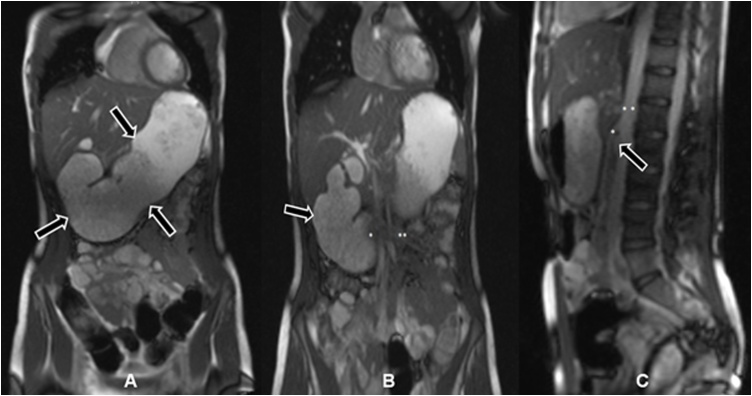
Abdominal MRI showing (A) a dilated stomach (arrows), (B) a dilated duodenum (arrow) with change in caliber from before (*) to after () under passing the mesenteric root. Further an duodenal obstruction (C) between the superior mesenteric artery (*) and the abdominal aorta () can be seen. This duodenal obstruction is caused by a aorto-mesenteric angle of 14°, which is compared to normal angle values of 40–55°. These findings are compatible with a Wilkie’s syndrome (superior mesenteric artery syndrome) diagnosis.
